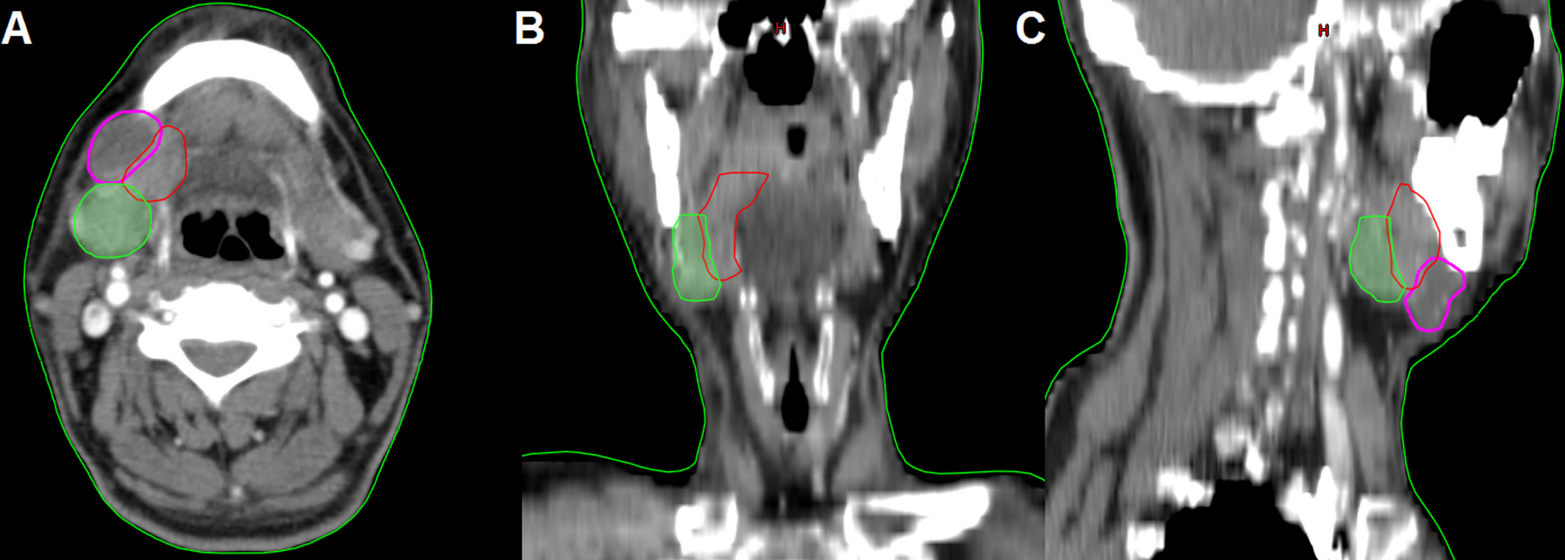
The preoperative computed tomography image of submandibular gland involvement in axial (A), coronal (B), and sagittal views (C) (red, primary tumor; purple, lymphadenopathy in level IB; green, submandibular gland).
